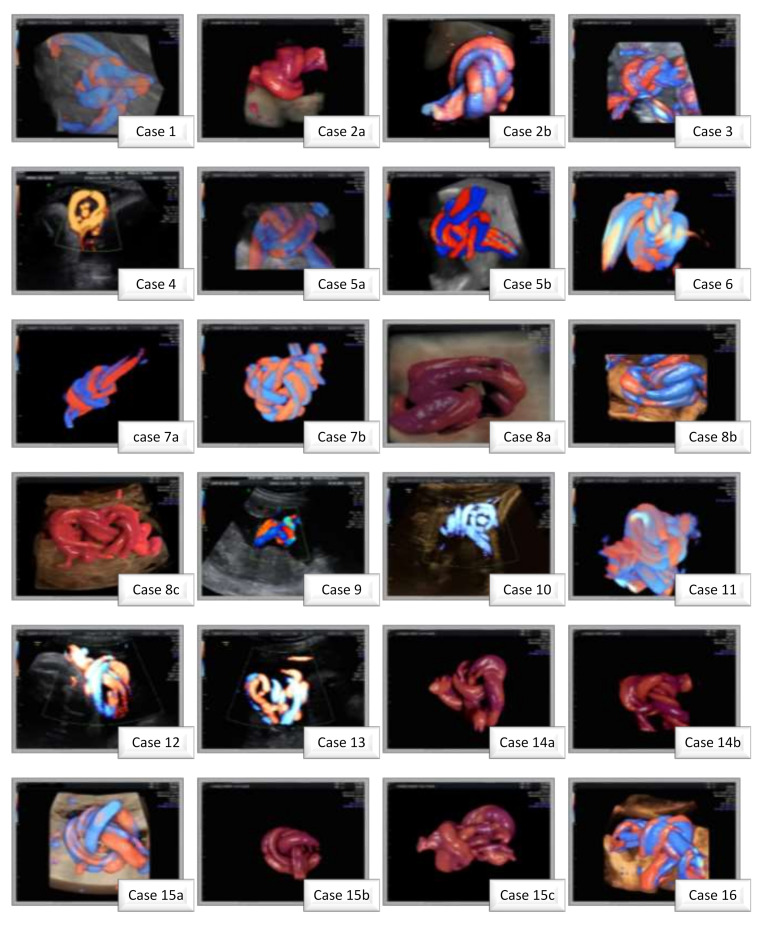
Power Doppler Ultrasound and and 3D-HD-Flow recontructed images of the prenataly diagnosed umbilical knots observed ofin the cases presented in this paper.
